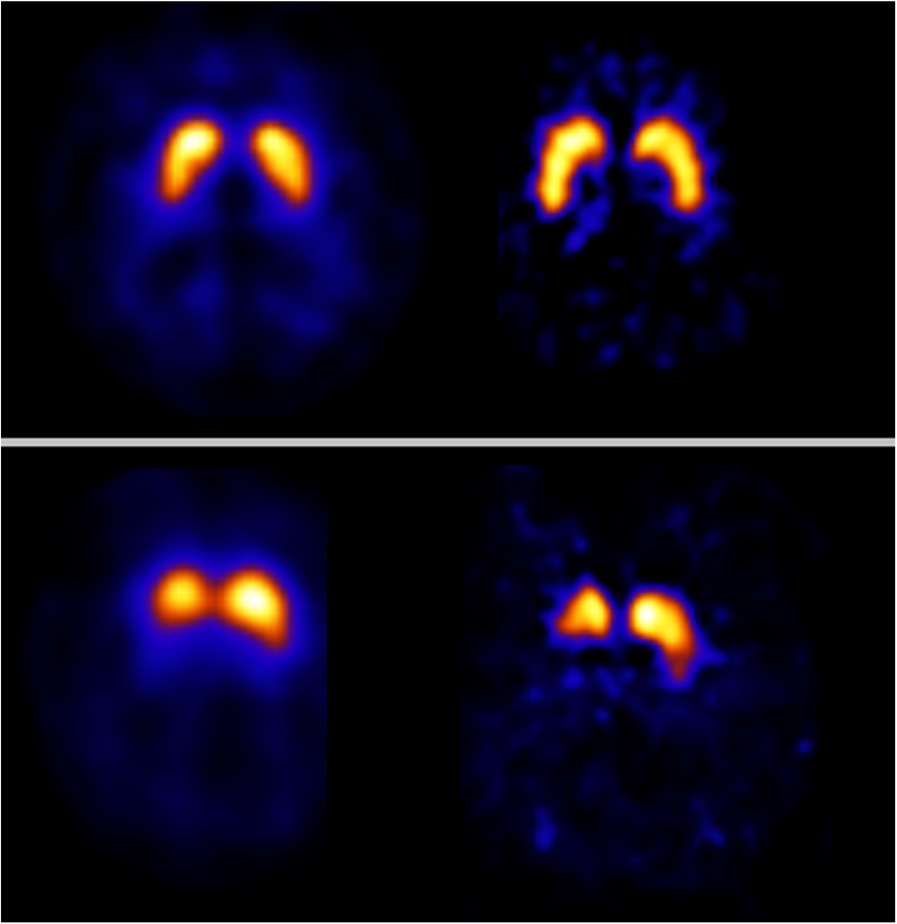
[^123^I]FP-CIT SPECT scans acquired on the NeuroFocus and InSPira. Representative image of a [^123^I]FP-CIT SPECT scan rated as “normal” (upper panel), and as abnormal (PD-like) (lower panel) on both systems. Images on the left and right were acquired on the NeuroFocus and InSPira, respectively. Images are displayed in radiological orientation (left is right)
